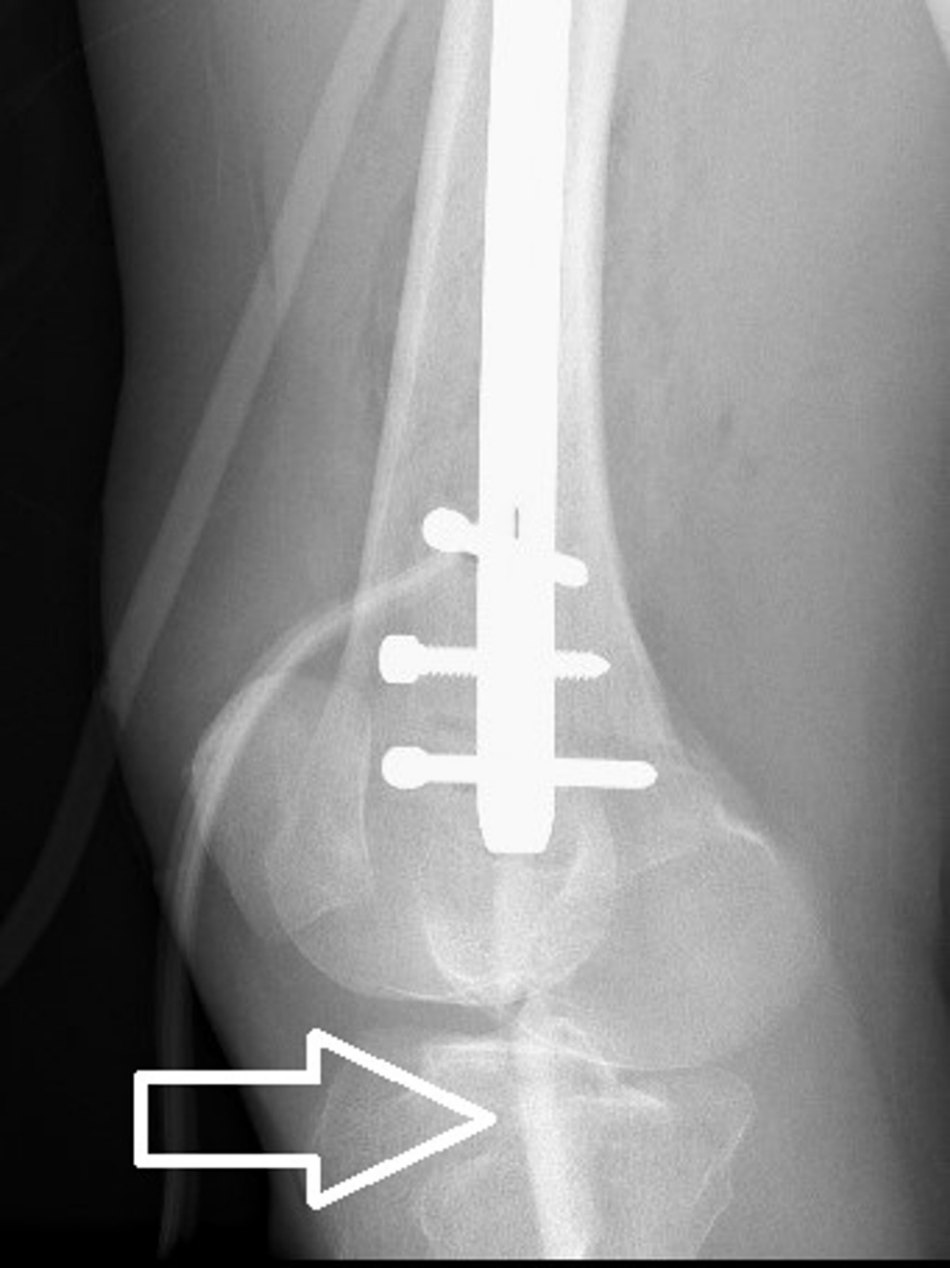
White arrow marks intraarticular bone fragment in lateral postoperative x-rays.
